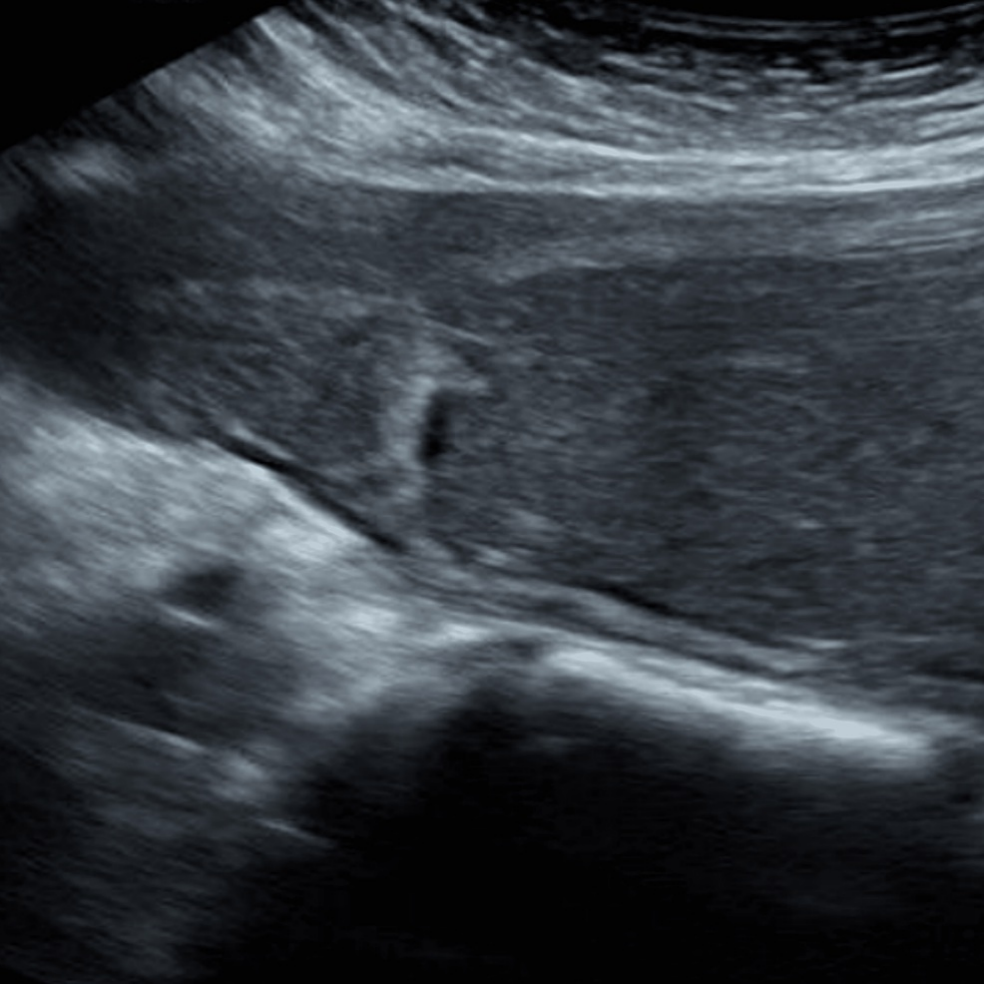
Ultrasonography revealed hematocolpos
